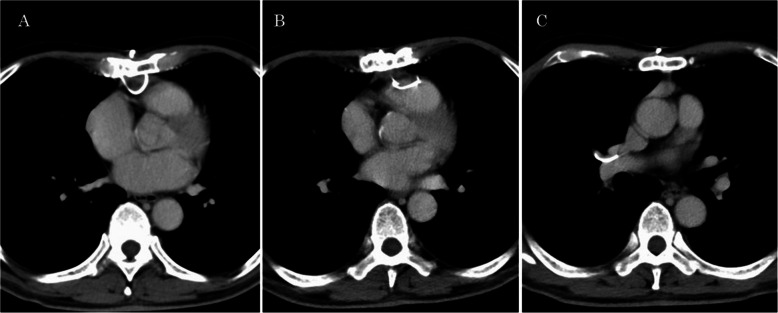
CT images performed after the initial operation. A Image after 6 months after the surgery. Redundant part of the sternal wire not securely fastened protrudes into the heart. B Image after 2 years. A fractured wire is observed in or on the right ventricle. C Image after 3 years. A fragment of the wire is in the right pulmonary artery
